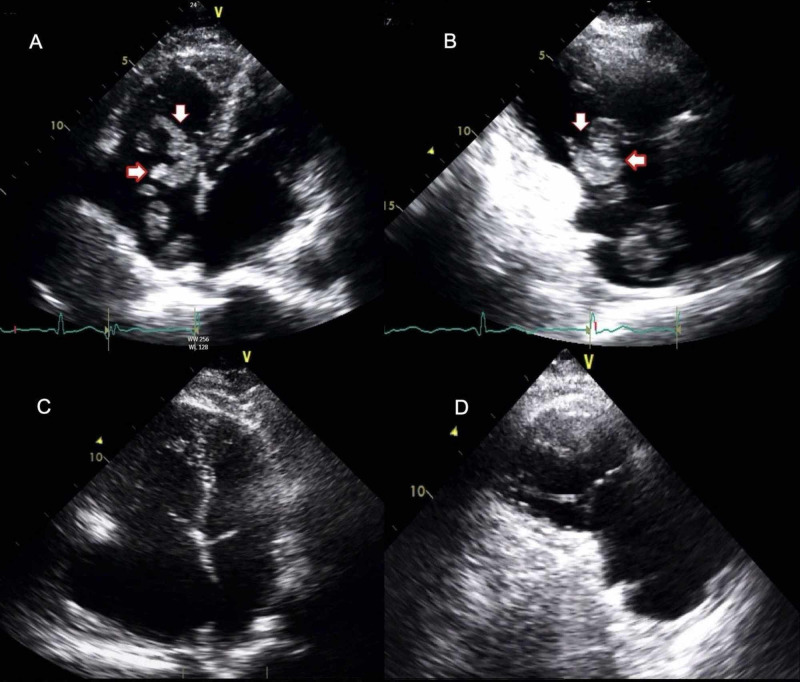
Transthoracic echocardiography of thrombus in transit Apical four-chamber view (A) and right ventricular inflow view (B) at admission, showing thrombus across the tricuspid valve (arrows). Apical four-chamber view (C) and right ventricular inflow view (D) after thrombolytic therapy showing the resolution of thrombus
